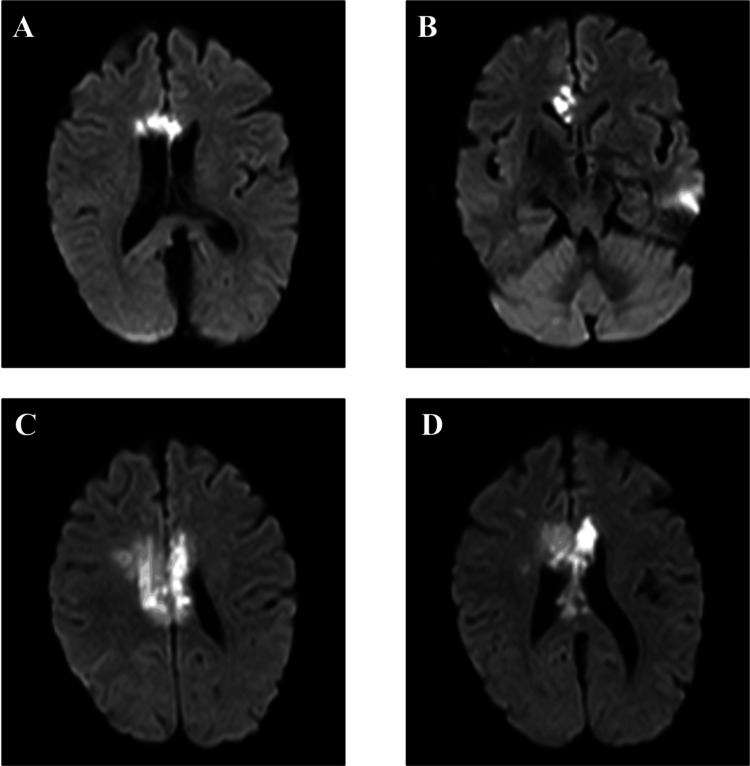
Repeat MRI showing new areas of callosal and pericallosal with high signal change bilaterally with restriction diffusion on DWI/ADC scans DWI/ADC: Diffusion-weighted imaging/apparent diffusion coefficient
